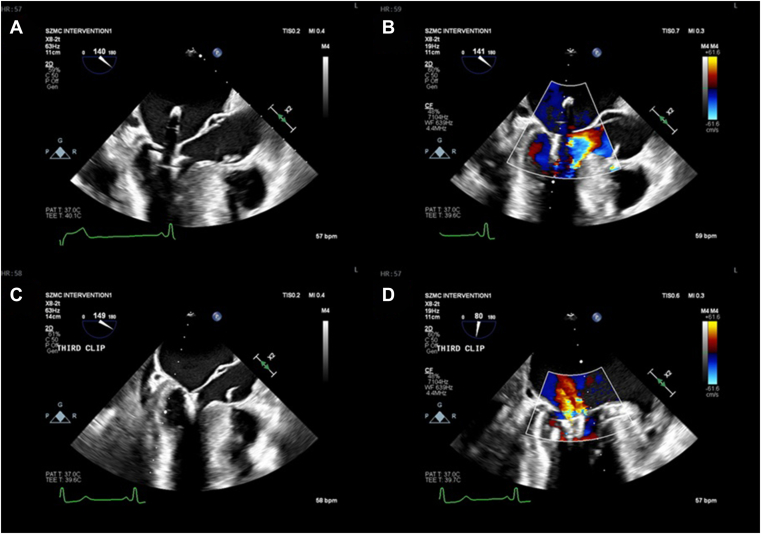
Transesophageal echocardiographic images obtained during and after the procedure. (A, B) During clip deployment, the device prevents systolic anterior motion of the mitral valve; (C, D) following clip implantation, a reduction in mitral regurgitation is demonstrated.
